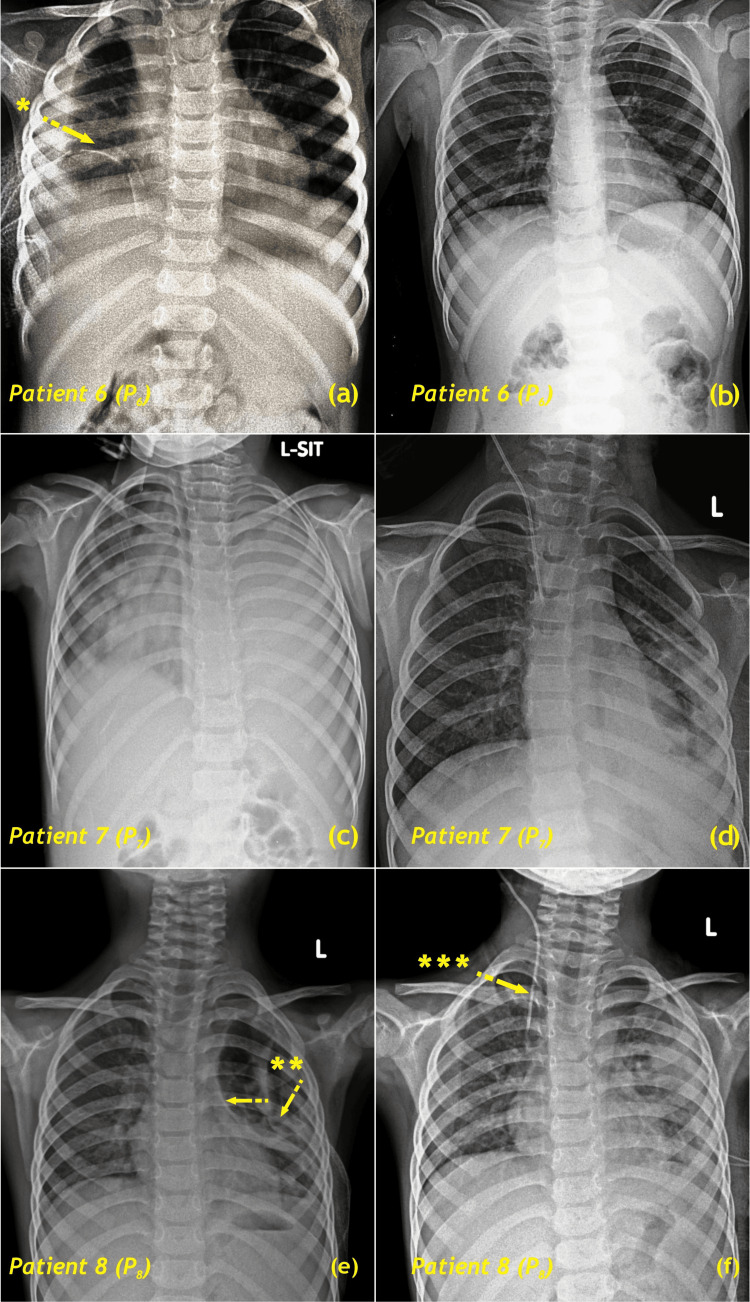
Chest radiographs pre- and postoperative Right decortication (P6), left decortication (P7, P8). (a, c, e) Preoperative; (b, d, f) postoperative. *, right intercostal drain; , loculated empyema; *, central venous pressure line Image credit: Vishal V. Bhende
